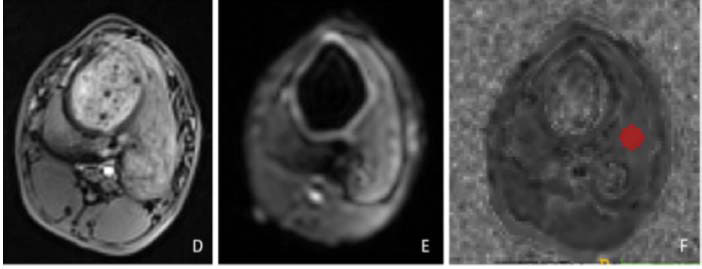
T1WI, DWI, and vMRE from left to right.
